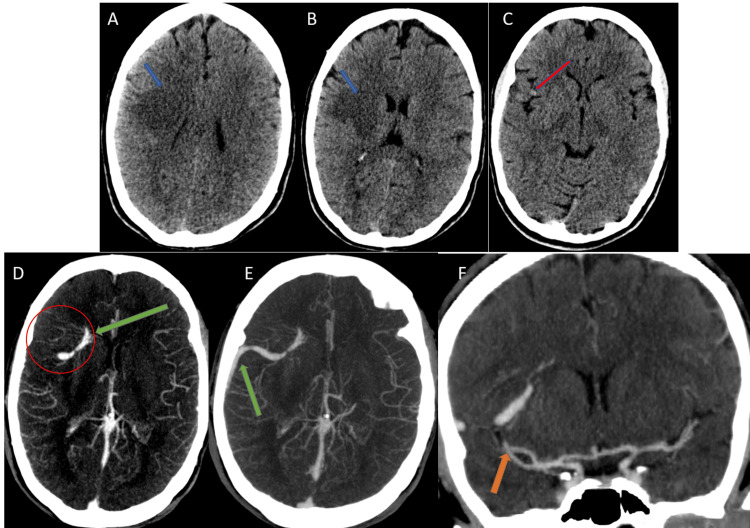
A CT scan of the brain and CT angiogram of a 25-year-old female patient who presented with acute left faciobrachial weakness of three hours duration. A plain CT scan of the brain (A, B, and C) showed an ill-defined area of hypoattenuation seen in the right frontal deep white matter (A, B) with effacement of the sulci. A hyperattenuating vascular structure was seen (C) in the right Sylvain fissure (arrows). A CT angiography performed five hours after the onset of symptoms (D, E, and F) showed a curvilinear-enhancing aberrant vascular structure extending from the right frontal horn to the Sylvain fissure and draining into the right transverse sinus (arrow in D and E). A few abnormal medullary veins draining into the beginning of this vessel were also noted (D, E, and F). Normal M1 and M2 segments of the right MCA were noted (F) (arrow).
